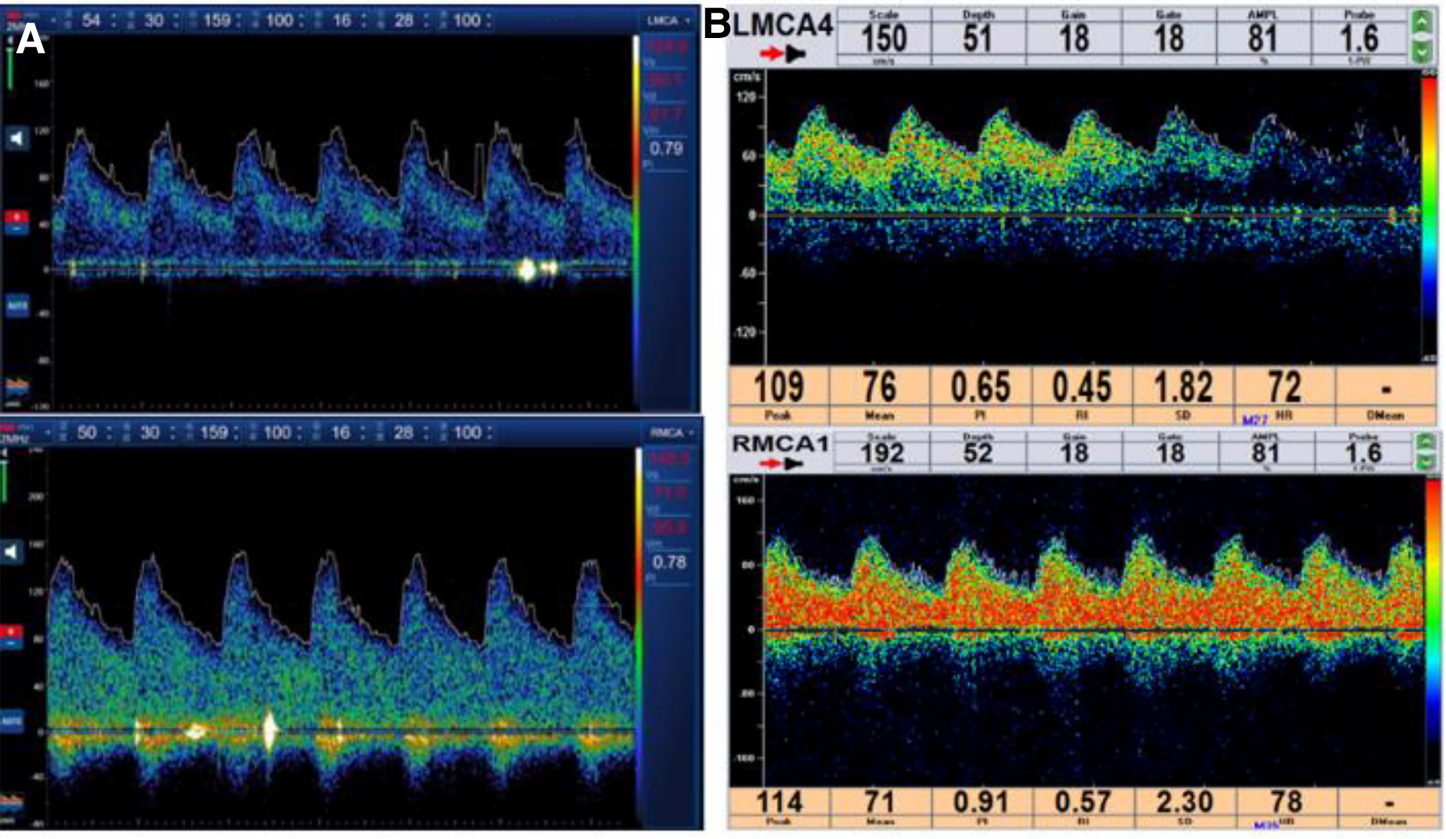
(A) Spectrum results of the bilateral middle cerebral artery detected by TCD after CAS. (B) Spectrum results of the bilateral middle cerebral artery detected by TCD before the operation. TCD, transcranial Doppler ultrasound; CAS, carotid artery stent implantation.
